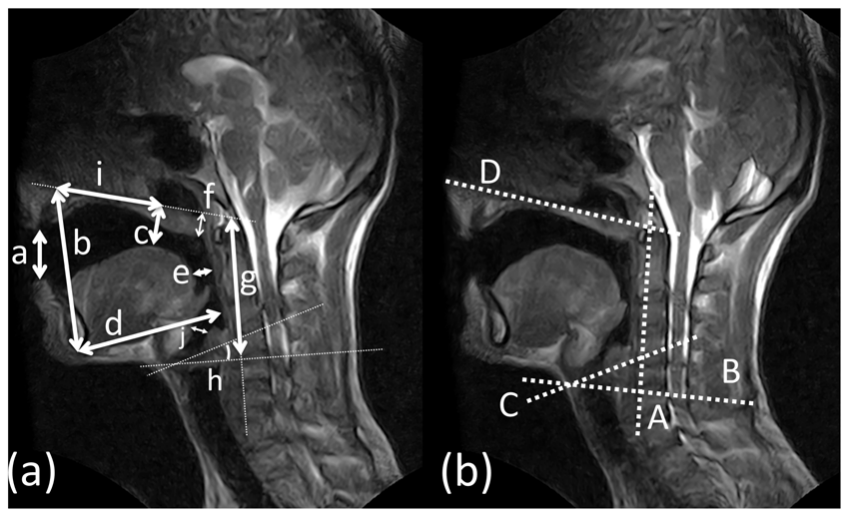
Vocal tract measurements. MR images of the vocal tract in sagittal orientation of a subject in the supine (a) and upright (b) position, using a balanced Steady State Free Precession Sequence. The subject held the note G4 on vowel/a/. Anatomical distance measurements are displayed with white lines and annotations. The dotted auxiliary lines are marked (A-D); measured distances are displayed by white lines with arrows and marked by small letters: a  =  lip opening, b  =  jaw opening, c  =  distance between the tongue and the jaw, d  =  jaw protrusion, e  =  oropharynx width, f  =  uvula elevation, g  =  larynx position, h  =  angle of larynx tilt, i  =  position of the highest point of the tongue (from the front) and j =  epilaryngeal tube. A more detailed explanation of the parameters measured can be found in the methods section.
